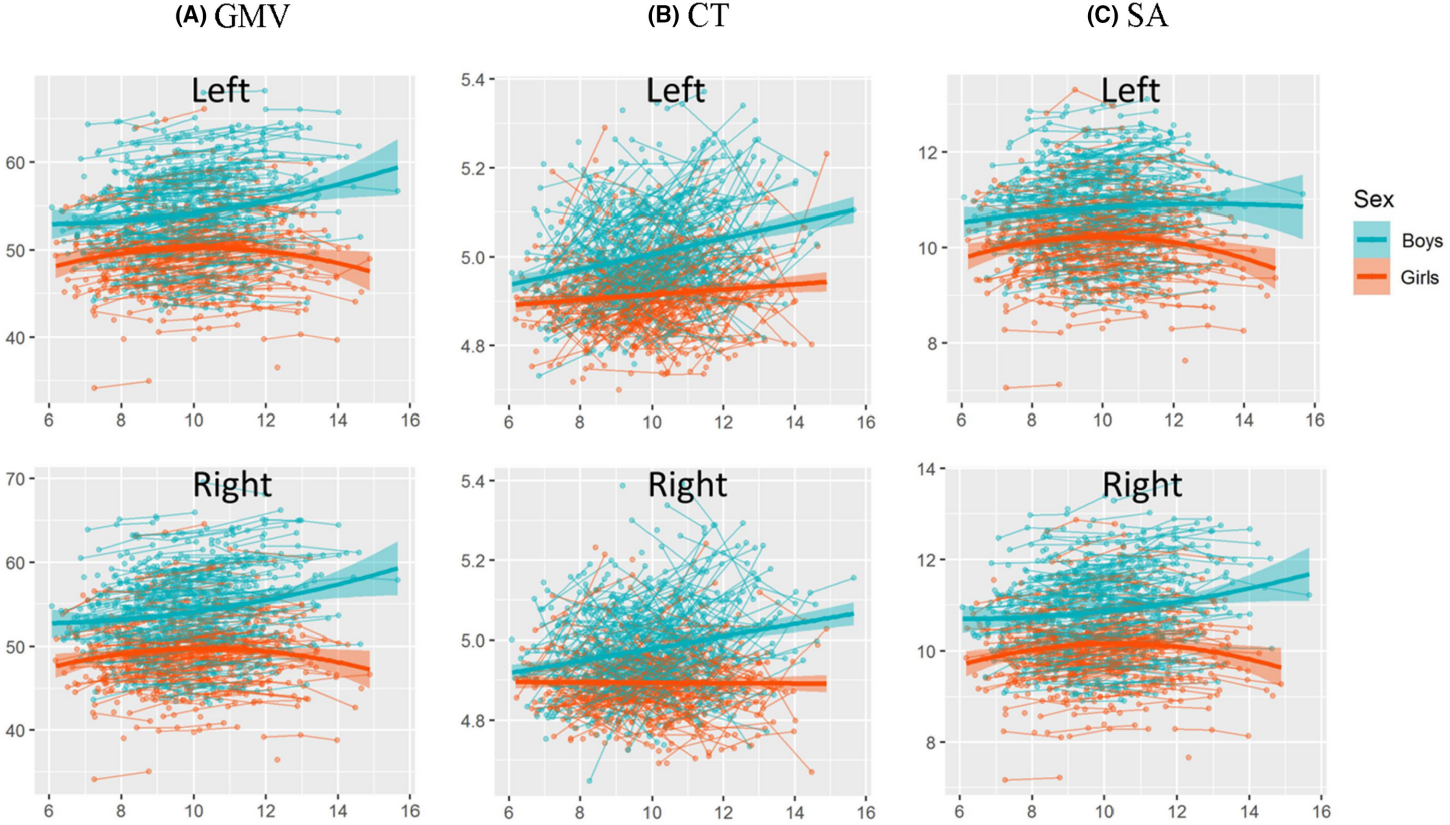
Development of the whole cerebellum. (A) GMV in cm^3^, (B) CT in cm, and (C) SA in cm^2^ (*y*‐axis) by age (*x*‐axis) and the optimal fitting model (quadratic, linear and quadratic) are shown. The shaded areas represent the 95% confidence intervals. Individual boys (blue) and girls (orange) are represented by individual lines, and participants measured once are represented by dots.
